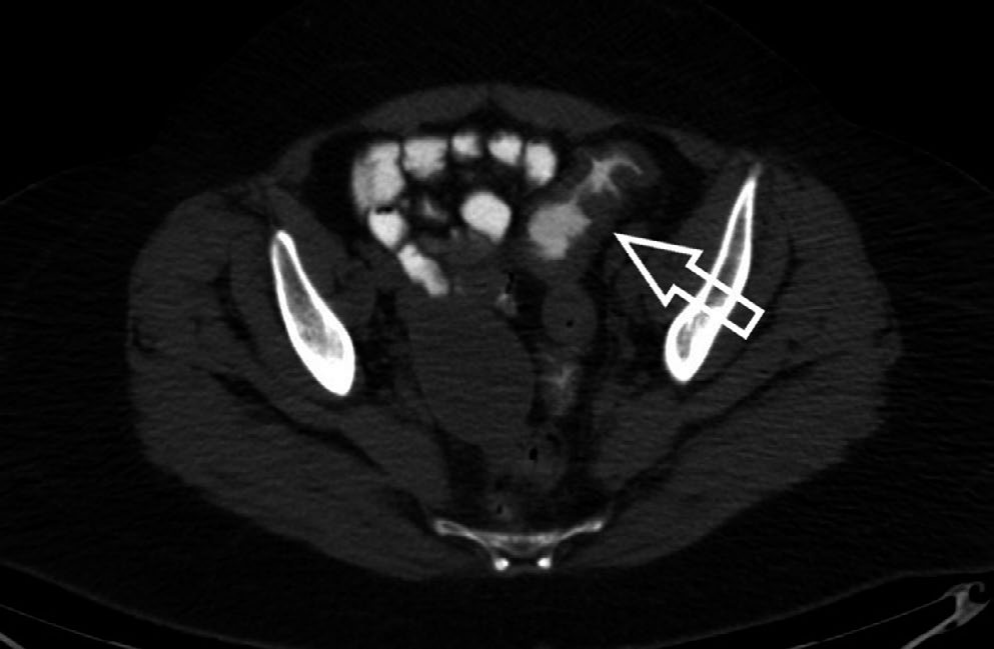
CT scan disclosed symmetric thickening of proximal sigmoid colon with a wall thickness of 2 cm (white arrow). It also detected a cystic lesion in the right ovary
